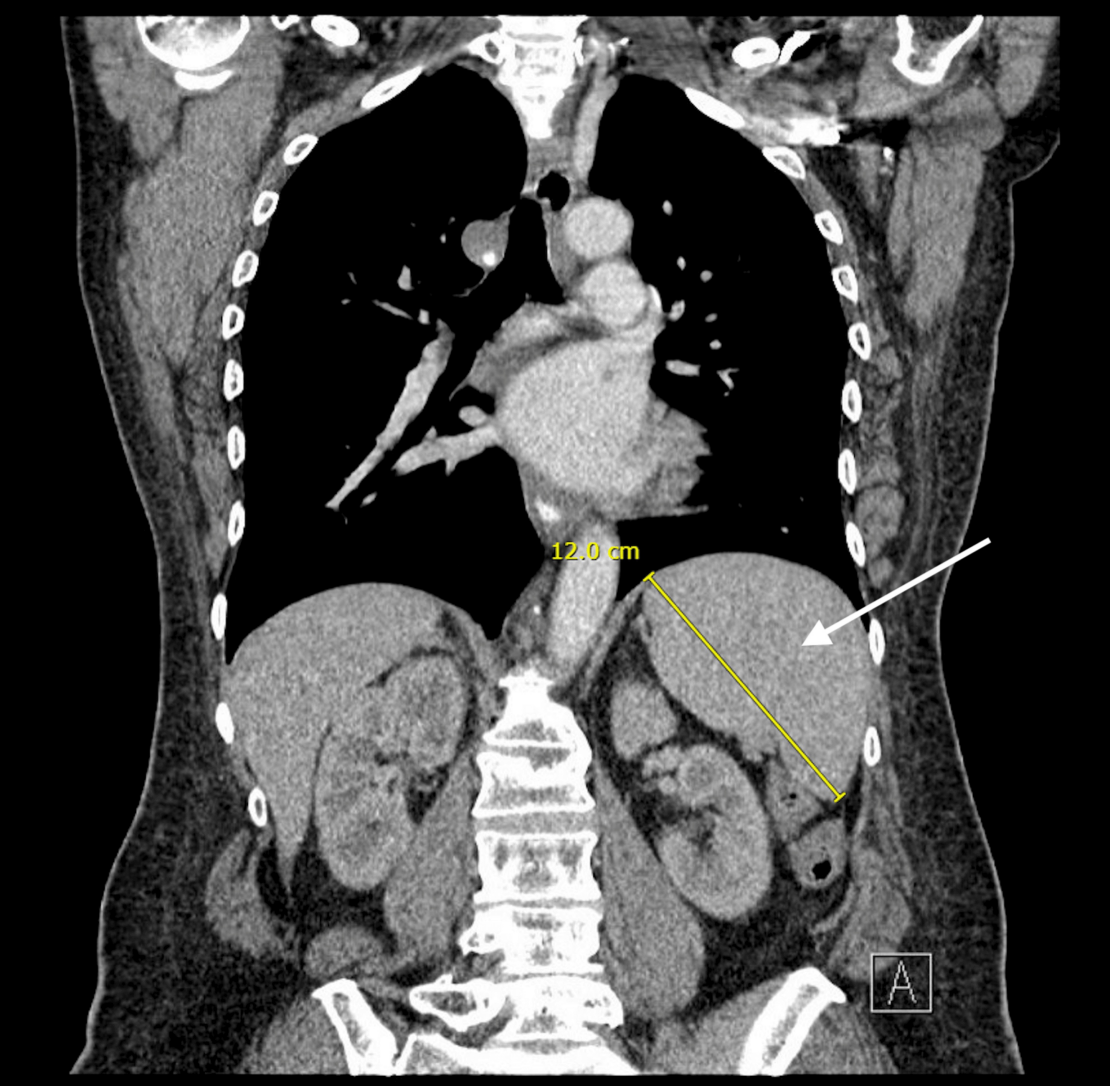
CT Scan Showing Borderline Splenomegaly CT abdomen/pelvis showing borderline splenomegaly of approximately 12 cm, a nonspecific finding but also one of the minor criteria for POEMS syndrome (organomegaly). CT: computed tomography; POEMS: polyneuropathy, organomegaly, endocrinopathy, monoclonal gammopathy, and skin changes.
